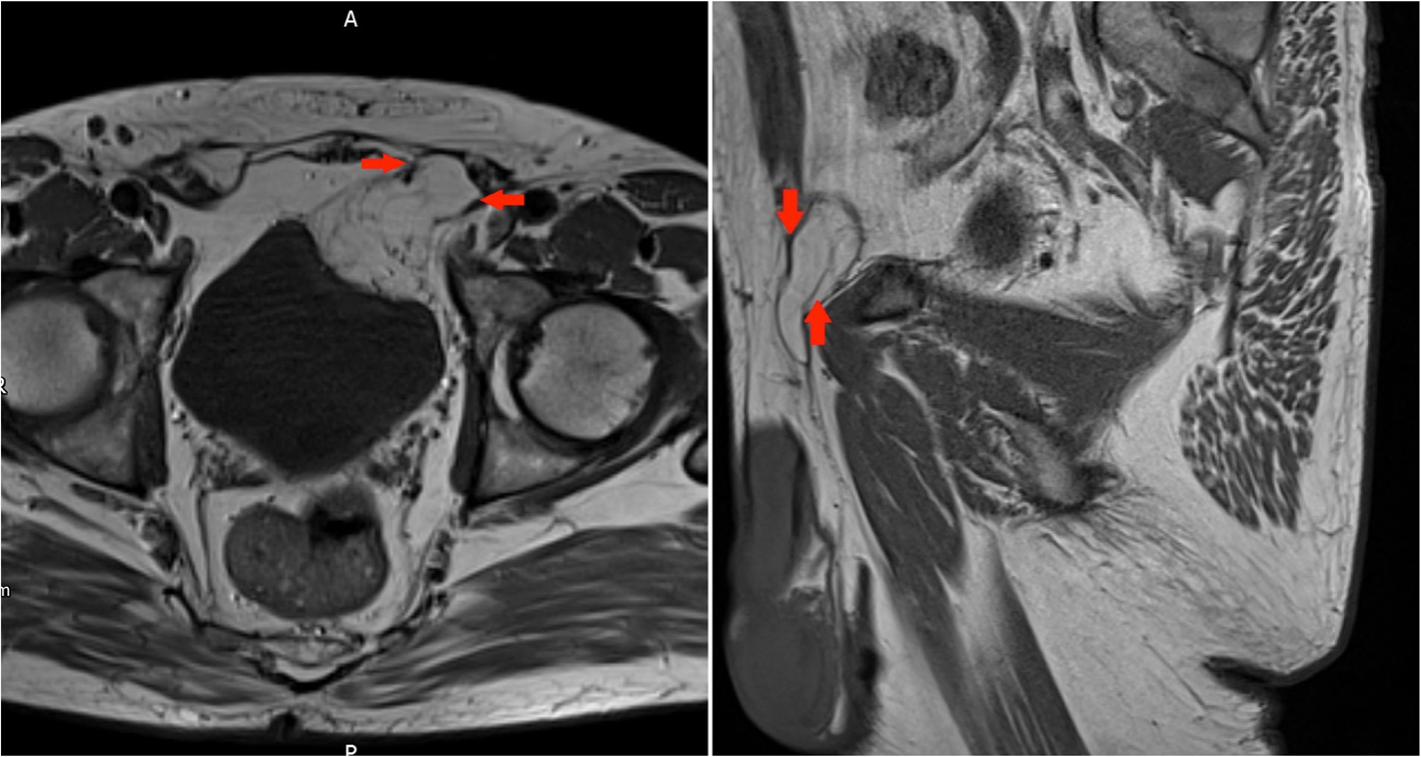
Left panel: Axial T1-weighted MR image of the pelvis: a lipomatous lesion arising within the pelvis and indenting the anterior bladder wall is seen with herniation (red arrows) medial to the epigastric vessels in keeping with a direct inguinal hernia. Right panel: Sagittal T1-weighted MR image of the pelvis: red arrows outline the neck of the direct inguinal hernia containing a lipomatous lesion. The hernial sac content is bright on T1 in keeping with fat, the presence of internal septations and ‘haziness’ of the fat content suggest pathology other than a simple lipomatous lesion
